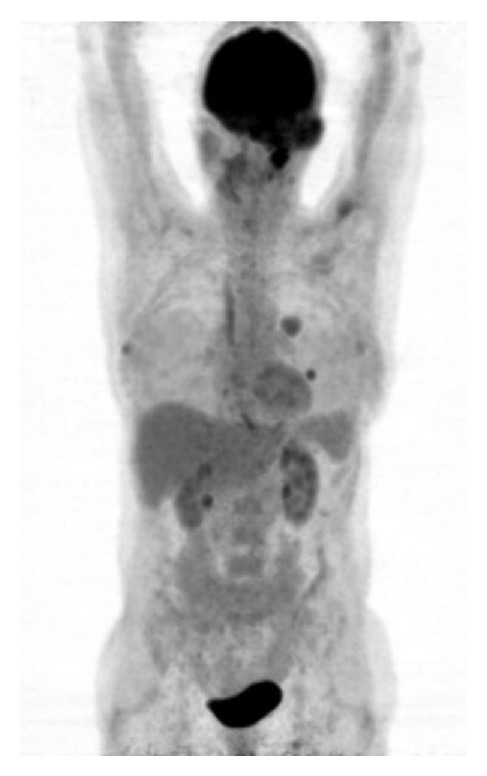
^18^F-FDG PET study in a patient with anaplastic meningioma. Increased FDG uptake in the primary tumor (left temporal region) as well as in its pulmonary metastases [67].
